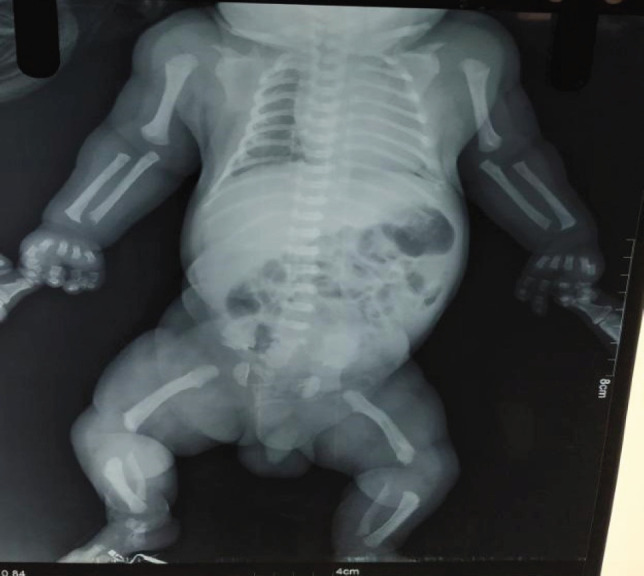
X-ray infantogram showing bilateral humerus and femur shortening.
